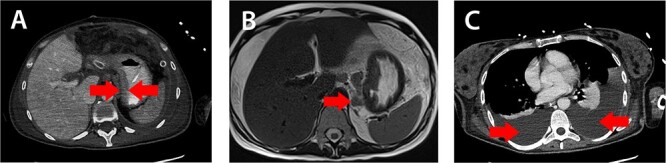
Magnetic resonance imaging: A) thickening of the gastric wall, B) perigastric lymph node enlargement and C) pleural effusion (red arrows).
